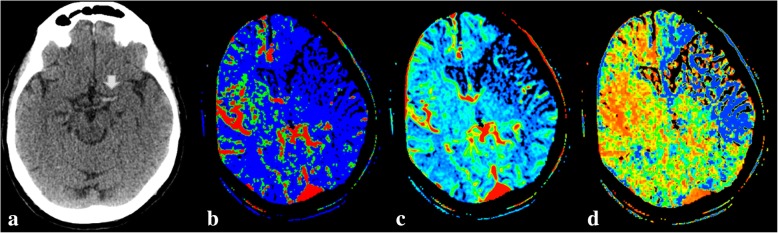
This 75-year-old woman presented with right hemiplegia and aphasia. Axial unenhanced CT shows a hyperdense vessel sign in the left MCA M1 segment (a, white arrow). CT perfusion was also performed, with parametric maps indicating CBF (b), CBV (c), and mean transit time (MTT) (d). These images reveal a large established core infarct with no significant penumbra. This indicates a complete stroke; no endovascular treatment was attempted
